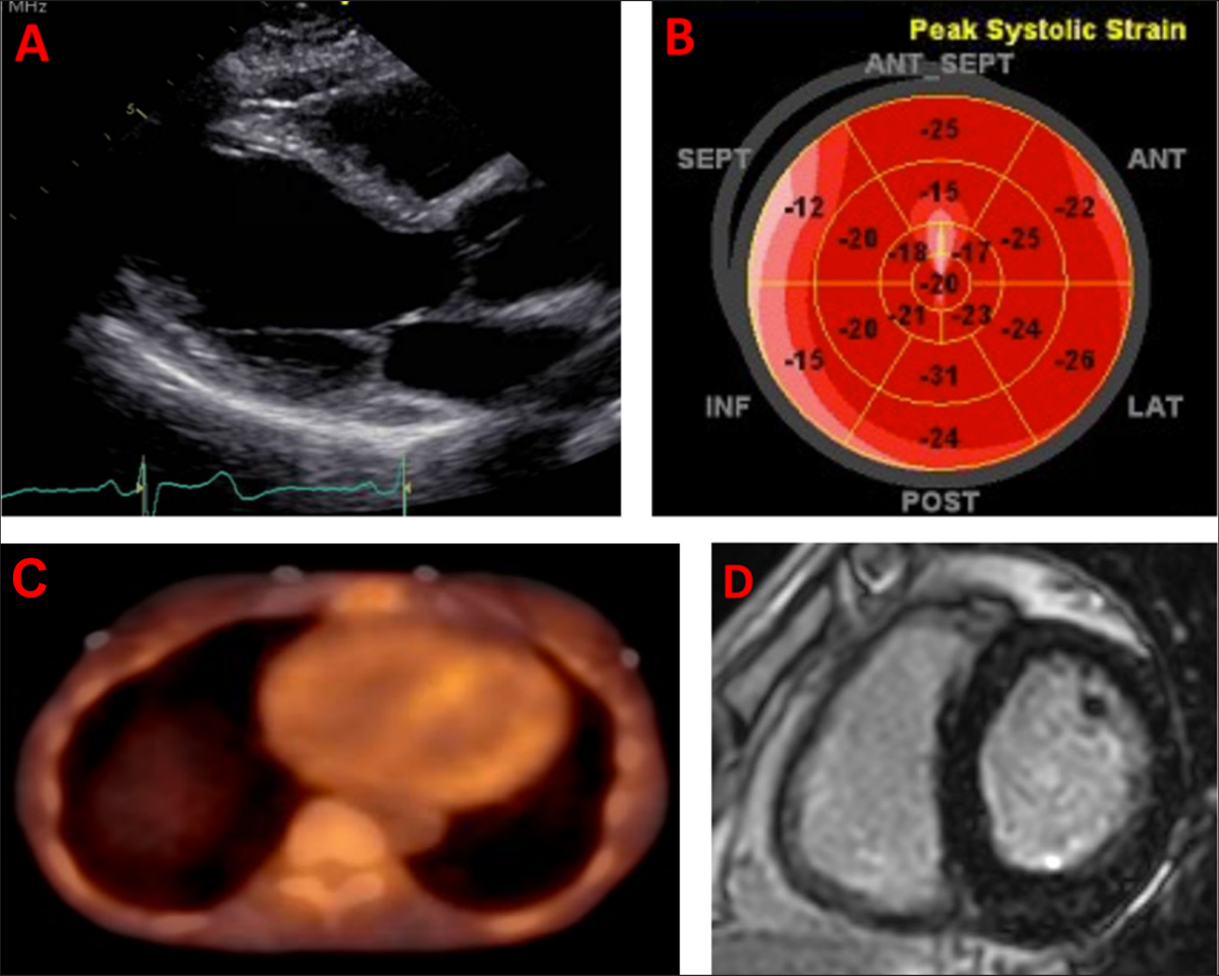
Multimodality imaging in the workup of a 70-year-old patient who is a carrier for the p.V50M variant and who presented with exertional shortness of breath and palpitations. (A) Echocardiogram parasternal long-axis window showing normal left ventricular wall thickness. (B) Depressed longitudinal strain, particularly in the basal septal segments (absence of apical sparing pattern). (C) 99m-technetium pyrophosphate single photo emission computed tomography showing diffuse uptake of the tracer in the myocardium. (D) Cardiac magnetic resonance imaging with phase-sensitive inversion recovery sequences obtained 15 minutes post gadolinium show minimal late gadolinium enhancement, but dedicated sequences showed elevated native T1 (1130 millisecond, 1.5 Tesla) and extracellular volume fraction (39%).
